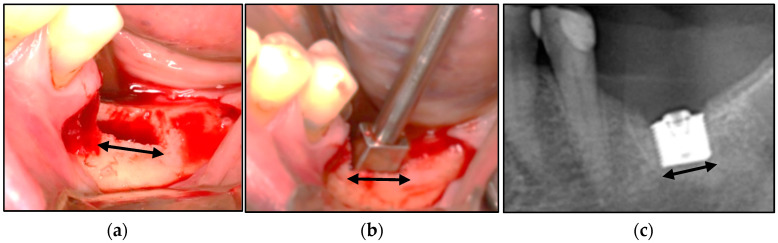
Patient 1. (a) The rectangular osteotomy. (b) The trial-fit gauge was tested repeatedly to achieve complete seating. (c) The RBI was inserted to full depth without bony fracture and achieved primary stability. This was visualised radiographically. Black arrows represent relative 6 mm lengths.
